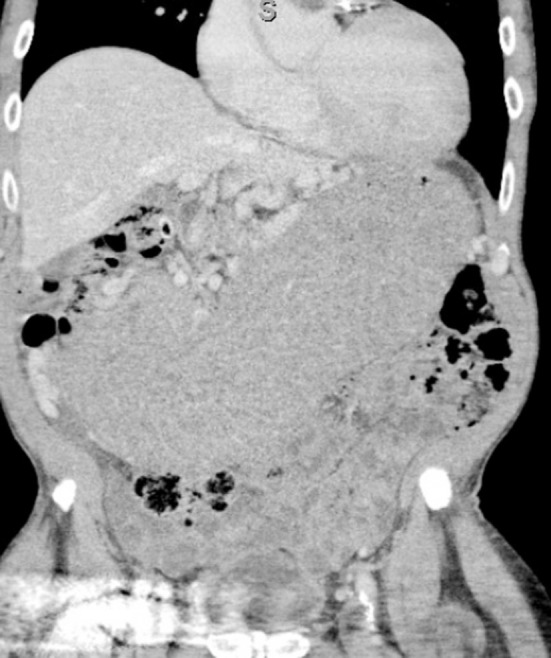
Computerised Tomogram (CT) -Abdomen; Coronal view, grossly enlarged Stomach.
